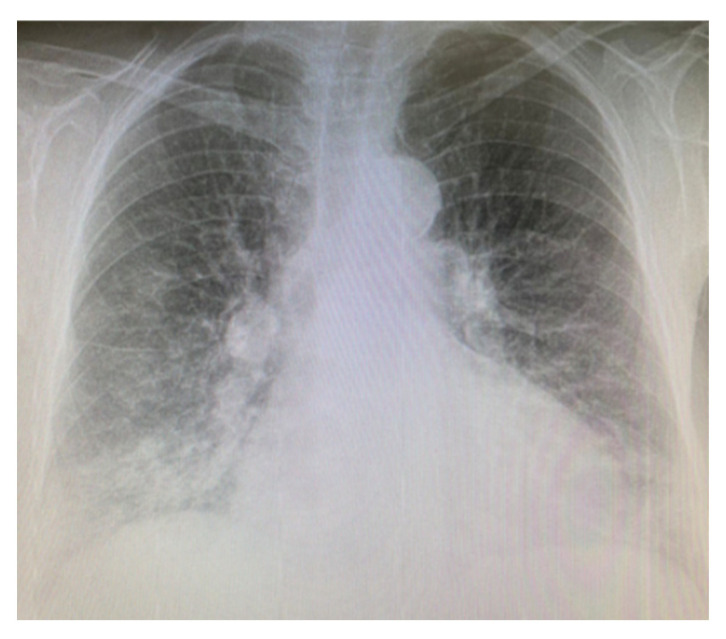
Chest X-ray—2017—Case 3. Nodular and micronodular opacities localized mainly in the lower lobes. Veiling of the pulmonary bases. Reticular opacities on both pulmonary areas. Enlarged cardiac silhouette.
